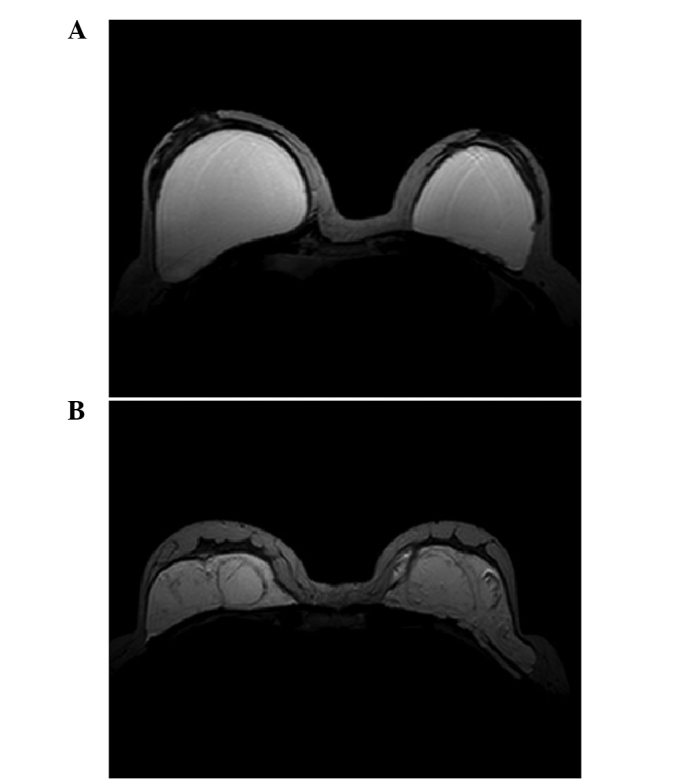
Axial T2-weighted MRI scans without fat depression of PAHG infiltrated in various layers. (A) Patient 8. PAHG was injected into the subglandular space and distributed regularly. (B) Patient 1. PAHG infiltrated into the surrounding tissue and was distributed irregularly. MRI, magnetic resonance imaging; PAHG, polyacrylamide hydrogel.
